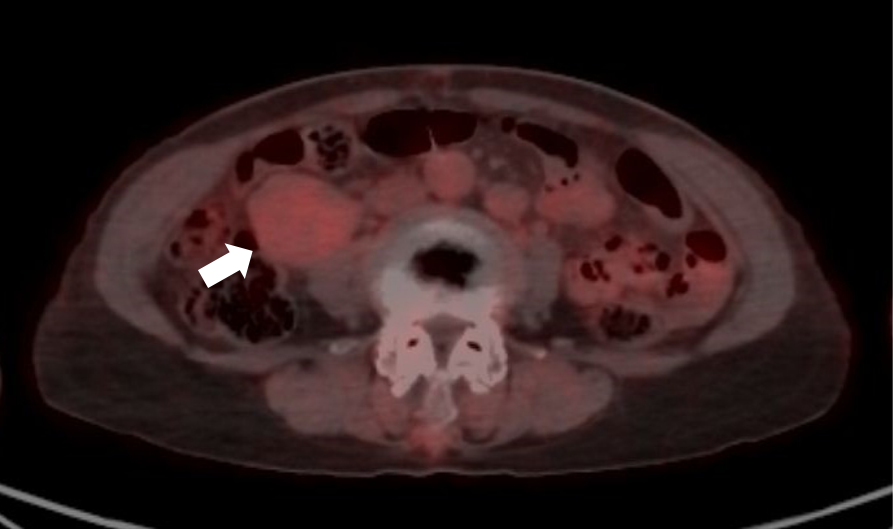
^18^F-fluorodeoxyglucose positron emission tomography (FDG-PET) of the leiomyosarcoma. Axial fusion images of FDG-PET revealing that the tumor (white arrows) had relatively low uptake
